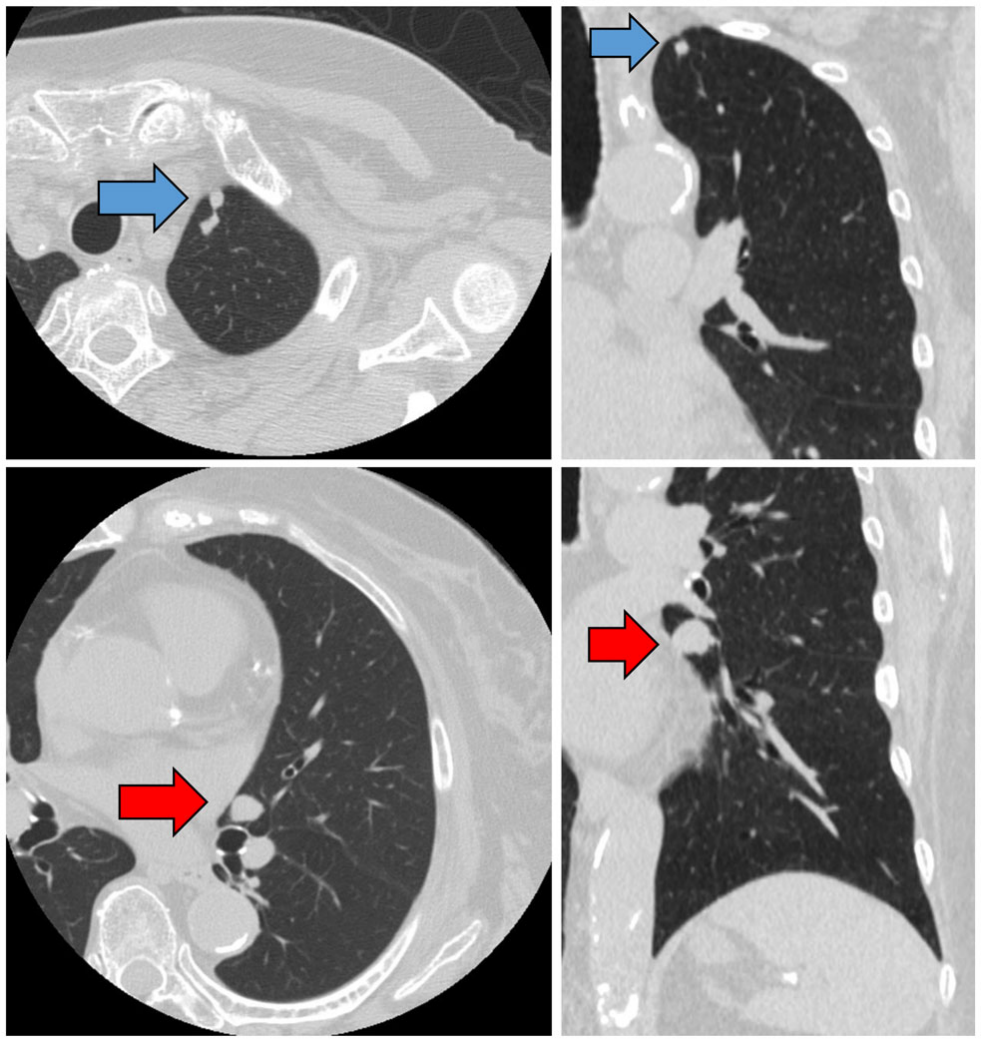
Computed tomography shows a 6‐mm nodule in the apex region (blue arrow) and a 15‐mm nodule in the lingual region (red arrows) of the left lung.
